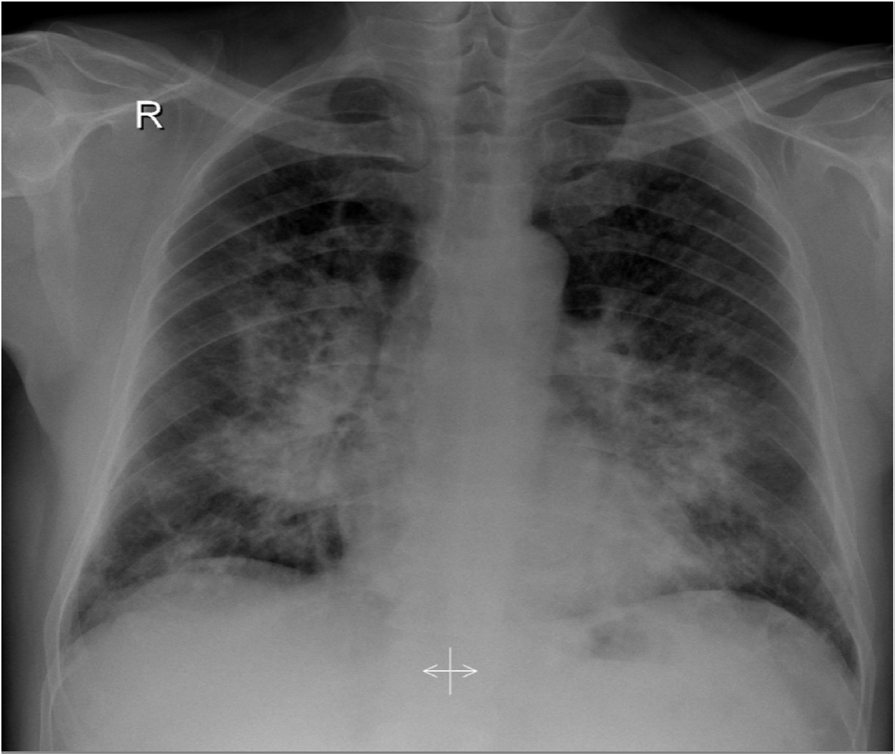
Chest X-ray showing bilateral patchy infiltration upon admission
